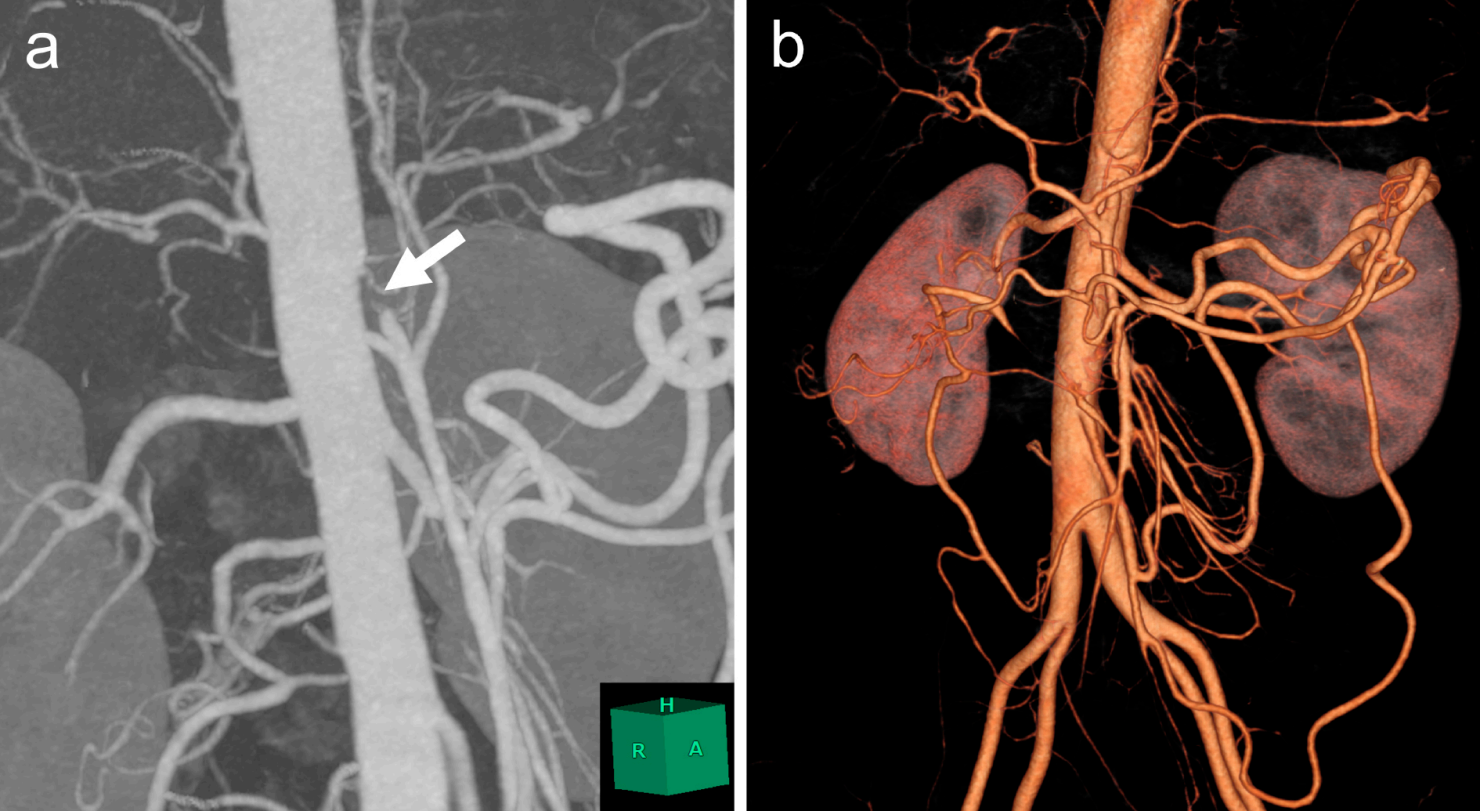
Computed tomography images showing occlusion of the common trunk of the celiac and superior mesenteric arteries (arrow) and the development of prominent collateral arteries via the inferior mesenteric artery. (a) Maximum intensity projection image. (b) Three-dimensional reconstruction image.
